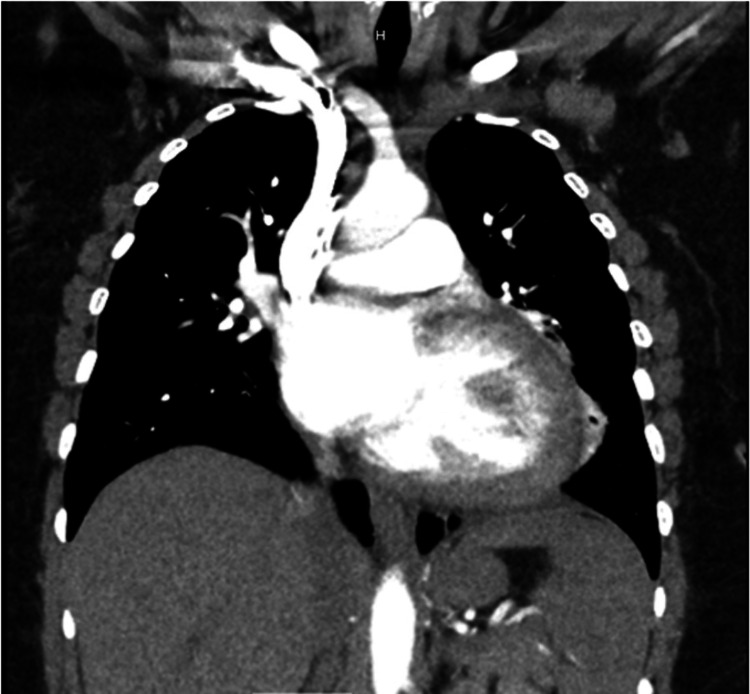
Chest CT scan (coronal view) This view of the chest CT scan demonstrated that the patient has cardiomegaly.
